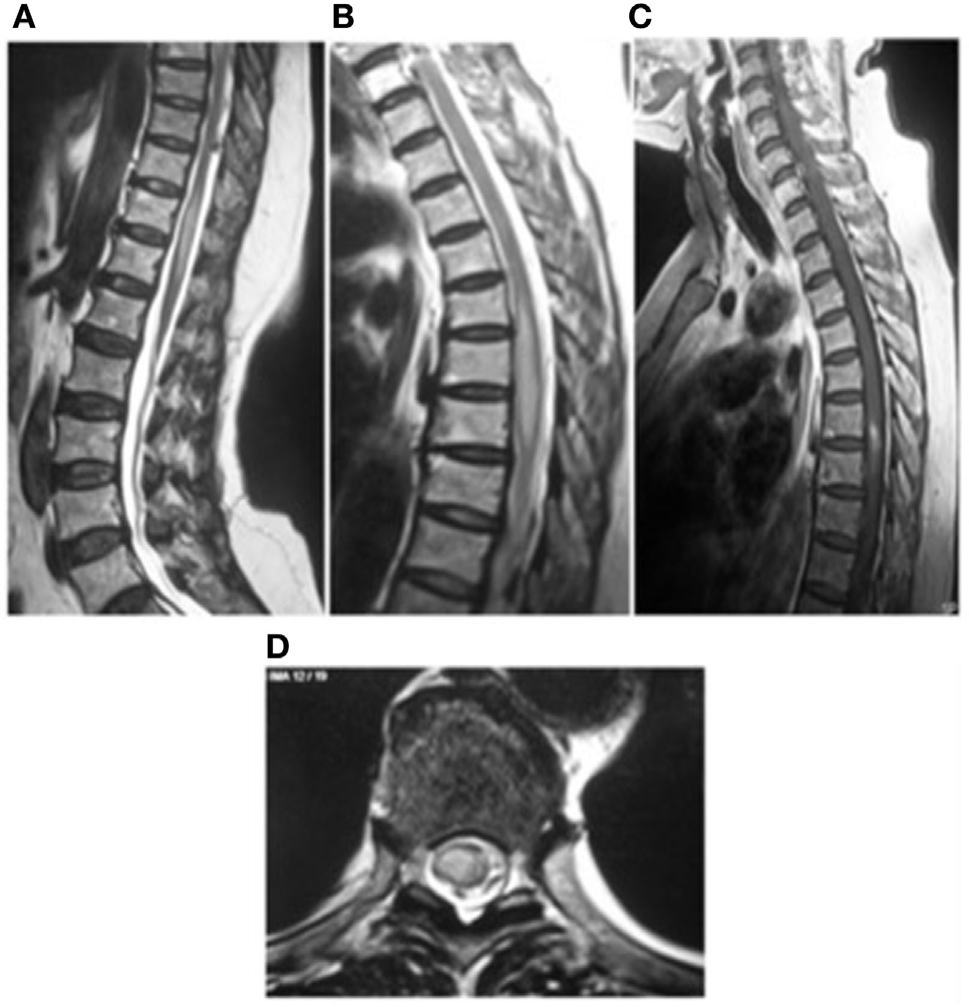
Myelitis (21) (A,B) sagittal T2-weighted image of spine showing high signal intensity from T5 to T7 and T10 to T12 levels. (C) T1-weighted images with contrast enhancement. (D) High signal intensity in the spinal cord in the sagittal images. This image has permissions for use.
